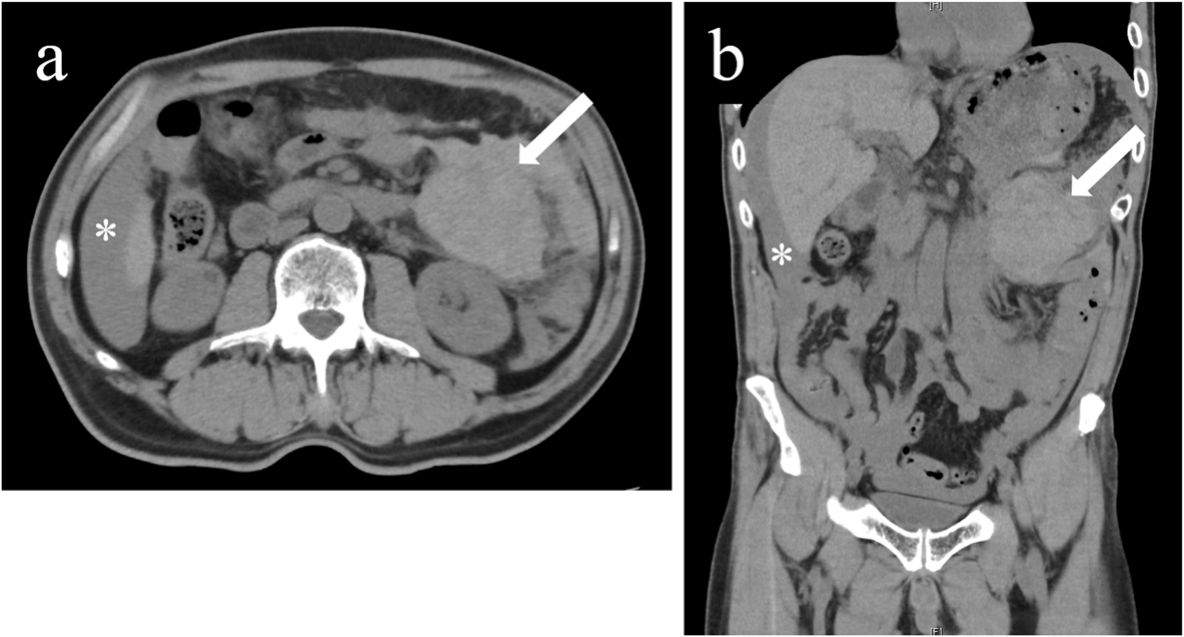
A computed tomography without contrast performed in the former hospital is shown; a an axial section and b a coronal section. A homogeneous mass lesion is located between the posterior wall of the stomach and the anterior side of the pancreatic tail (arrows) with intra-abdominal fluid collection (asterisks)
